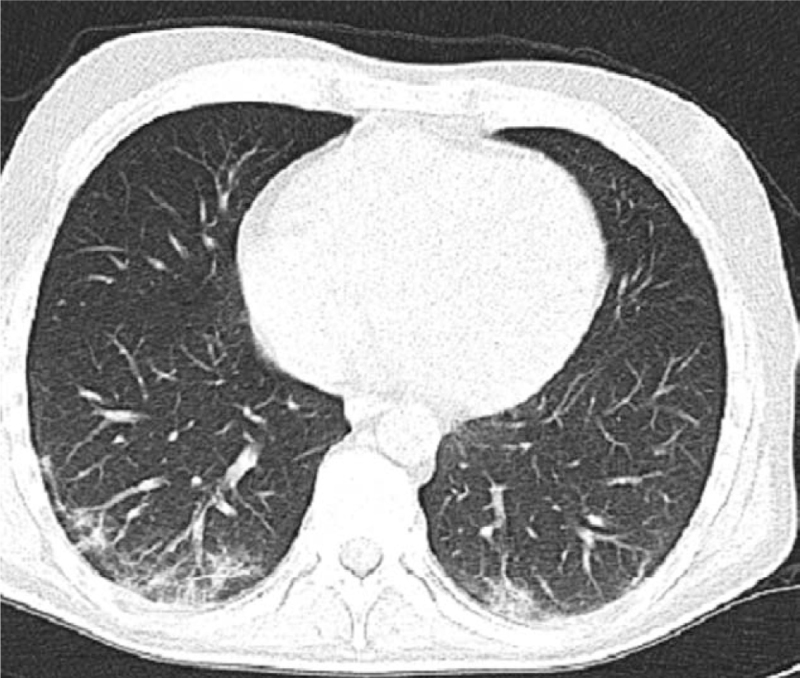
CT shows patchy ground-glass opacities affecting the subpleural lung parenchyma bilaterally, indicating interstitial pneumonia.
